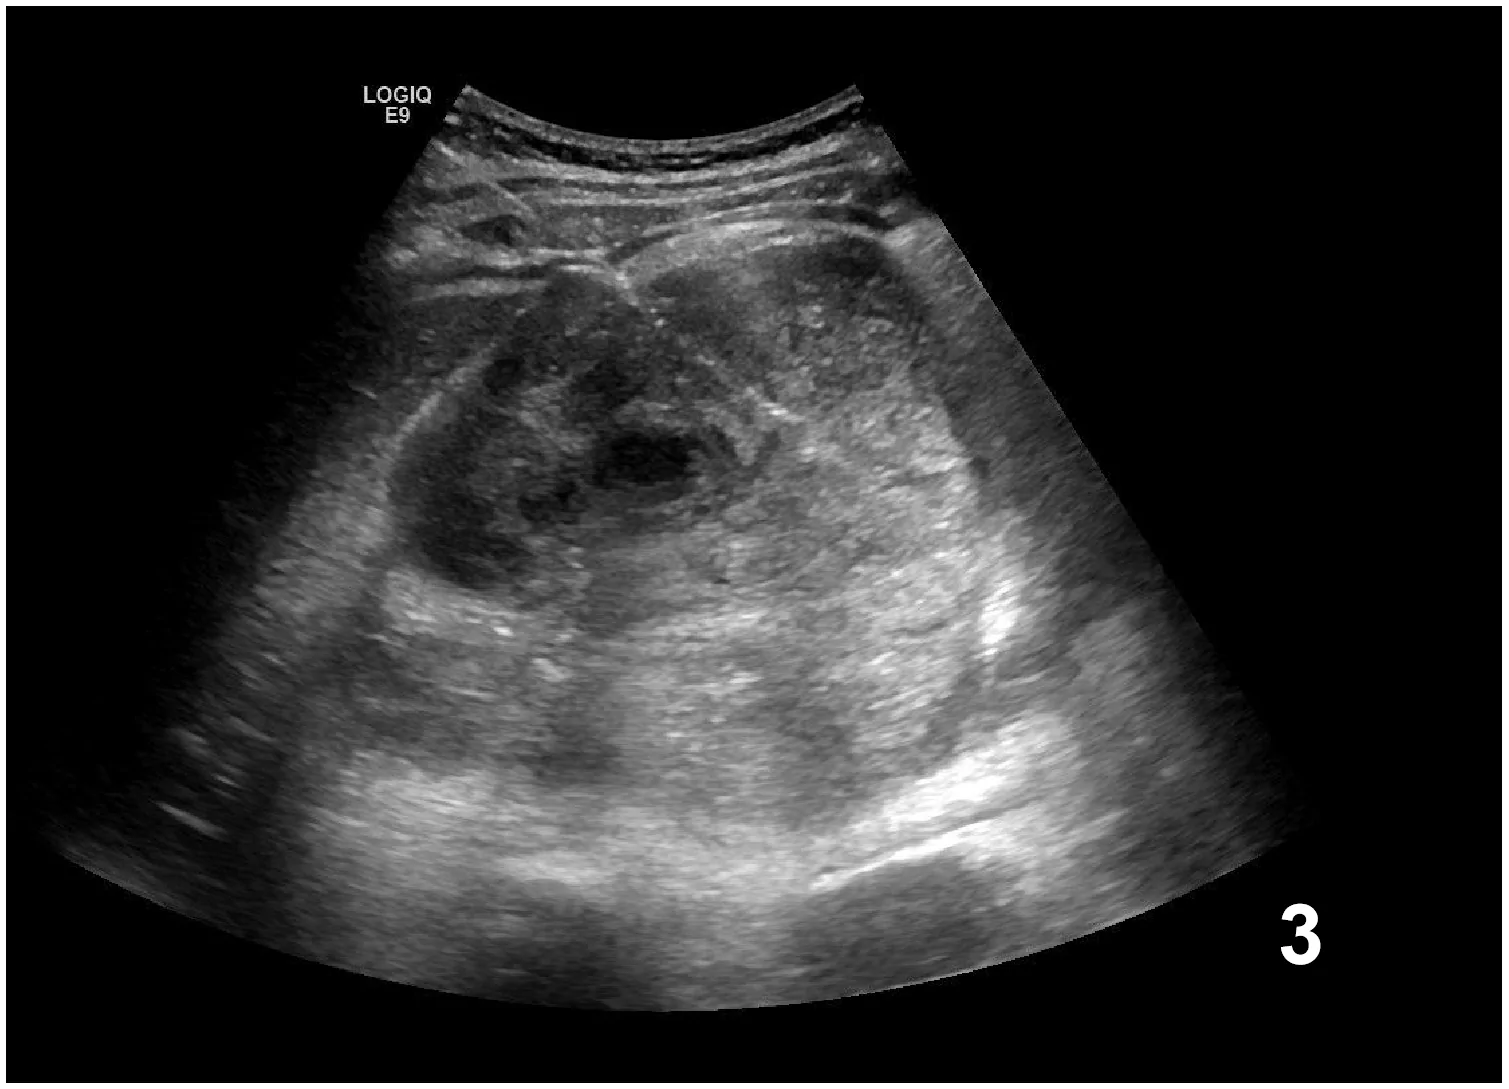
Male, 56 years old. Ewing-like undifferentiated small round cell neoplasm of the kidney. Ultrasound-guided biopsy.
